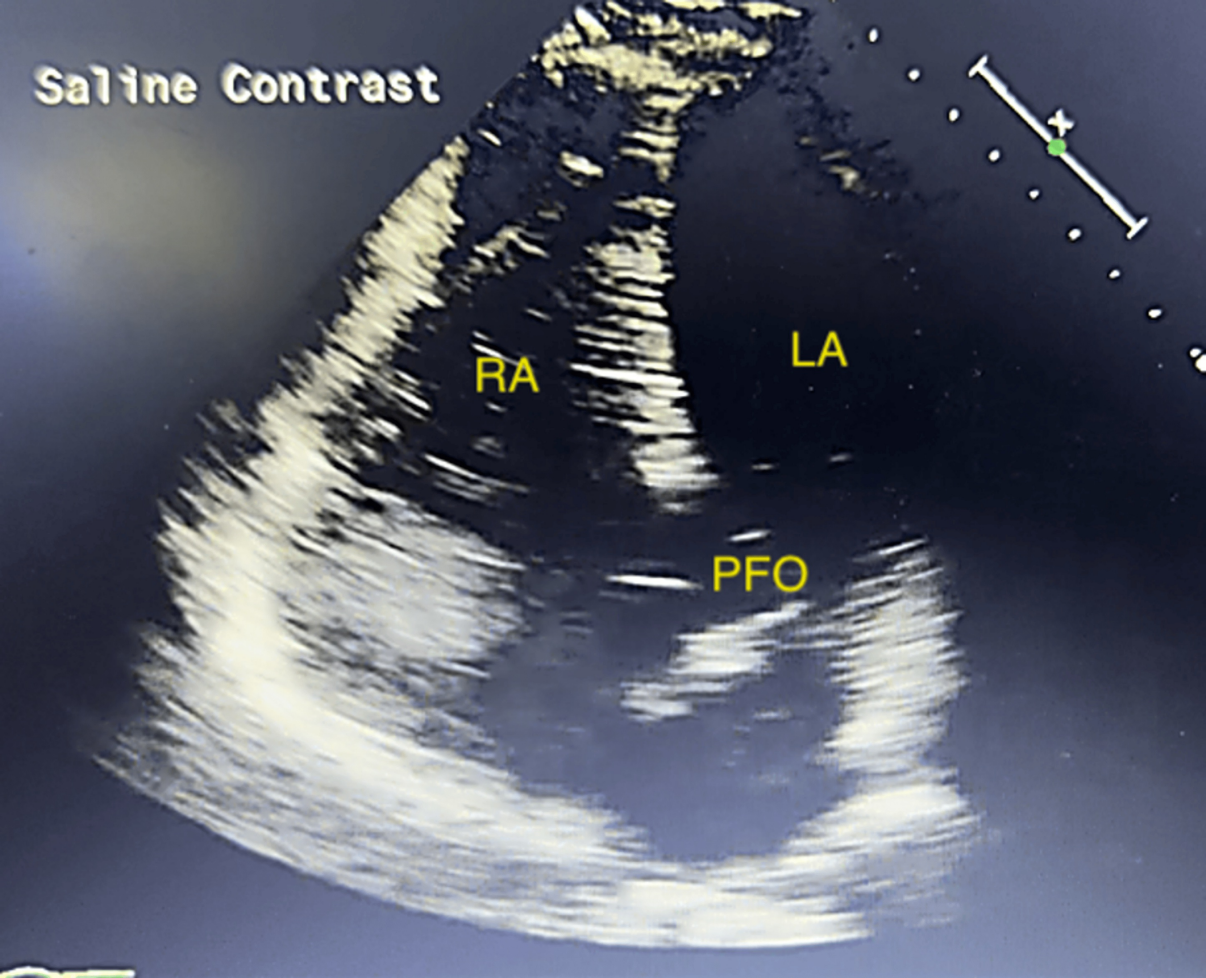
Transthoracic echo with bubble study showing contrast shunting through the patent foramen ovale
